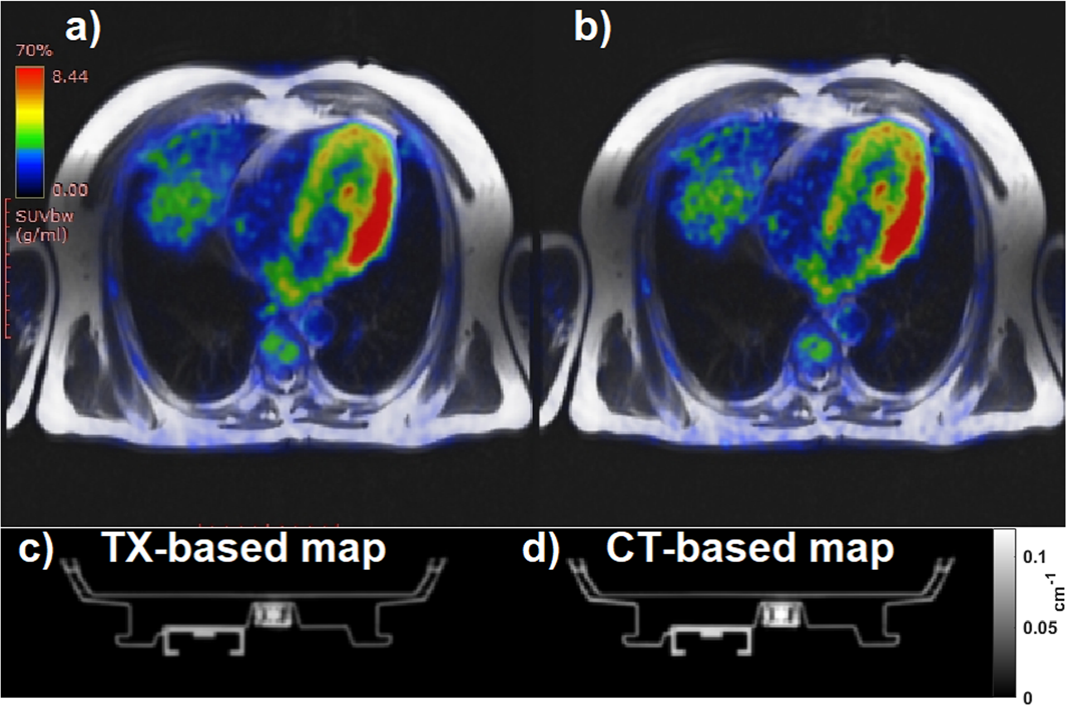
Fused images (a and b top) of the 2D-HASTE MRI to 18F-FDG PET for a volunteer. a The offline reconstructed PET with the scanner using hardware TX-based maps (both, patient table and posterior part of the array). b The same PET data, reconstructed using hardware CT-based maps. The lower row of the figure (c and d) shows, respectively, the TX-based and the CT-based maps of the patient table
